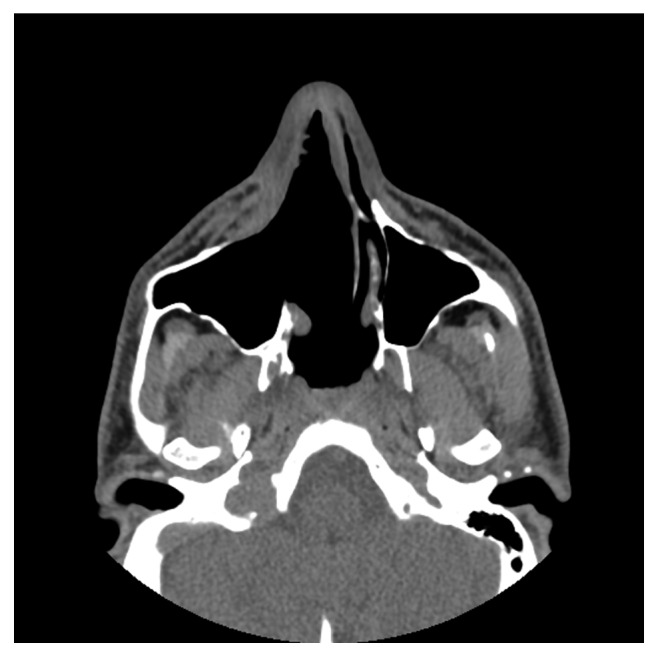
Postoperative CT, axial plane.
